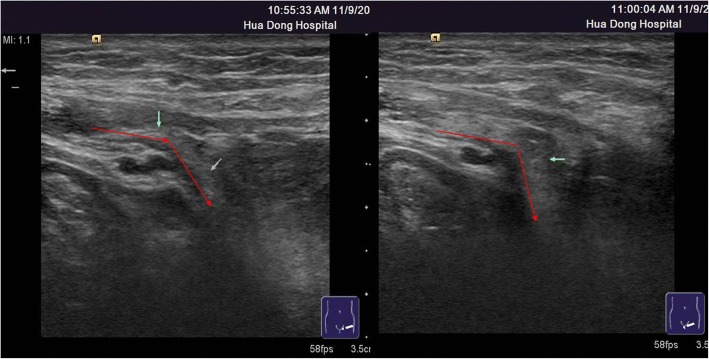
The spermatic cord showed in the ultrasonography. The spermatic cord was relaxed in supine position(the left graphy). After the patient changed to standing position(the right graphy), the spermatic cord became tight and the bending angle of it was reduced, which indicated that the spermatic cord was adhered in the deep inguinal ring
